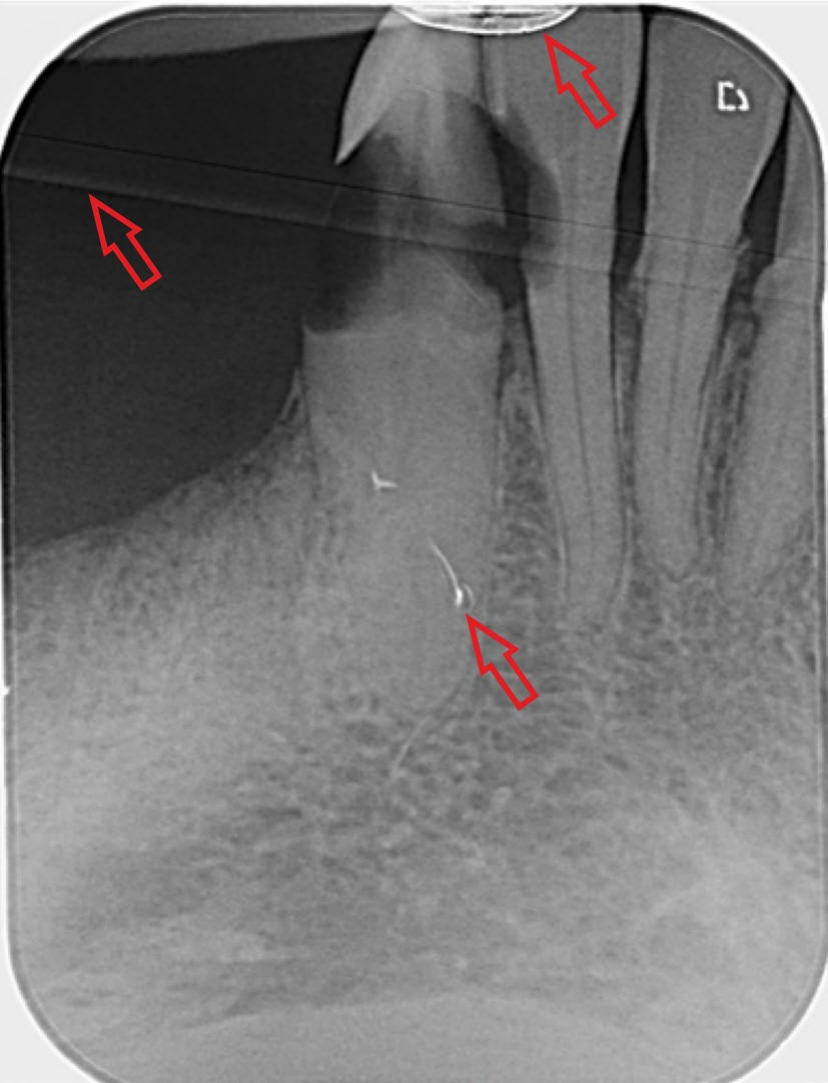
An example of a dental radiograph with quality defects of the image plate or PSP [scratches, folds and wear at the edge (see red arrows)], which are not relevant for the diagnosis [12, 15]. Findings: Teeth 42, 43 with carious destruction of the crown (tooth 43) and extensive caries on the distal aspect of the tooth (tooth 42). Secondary finding: Dental calculus on teeth 41 and 31. Widened periodontal space on tooth 41. [Colour figure can be viewed at wileyonlinelibrary.com]
